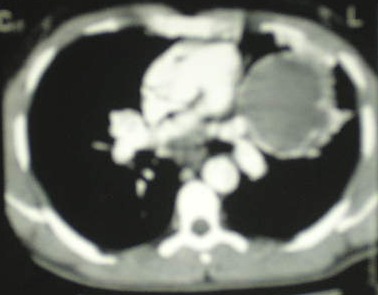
Chest's CT: a giant aneurysm in the left lower lobe pulmonary artery, with mural thrombus
